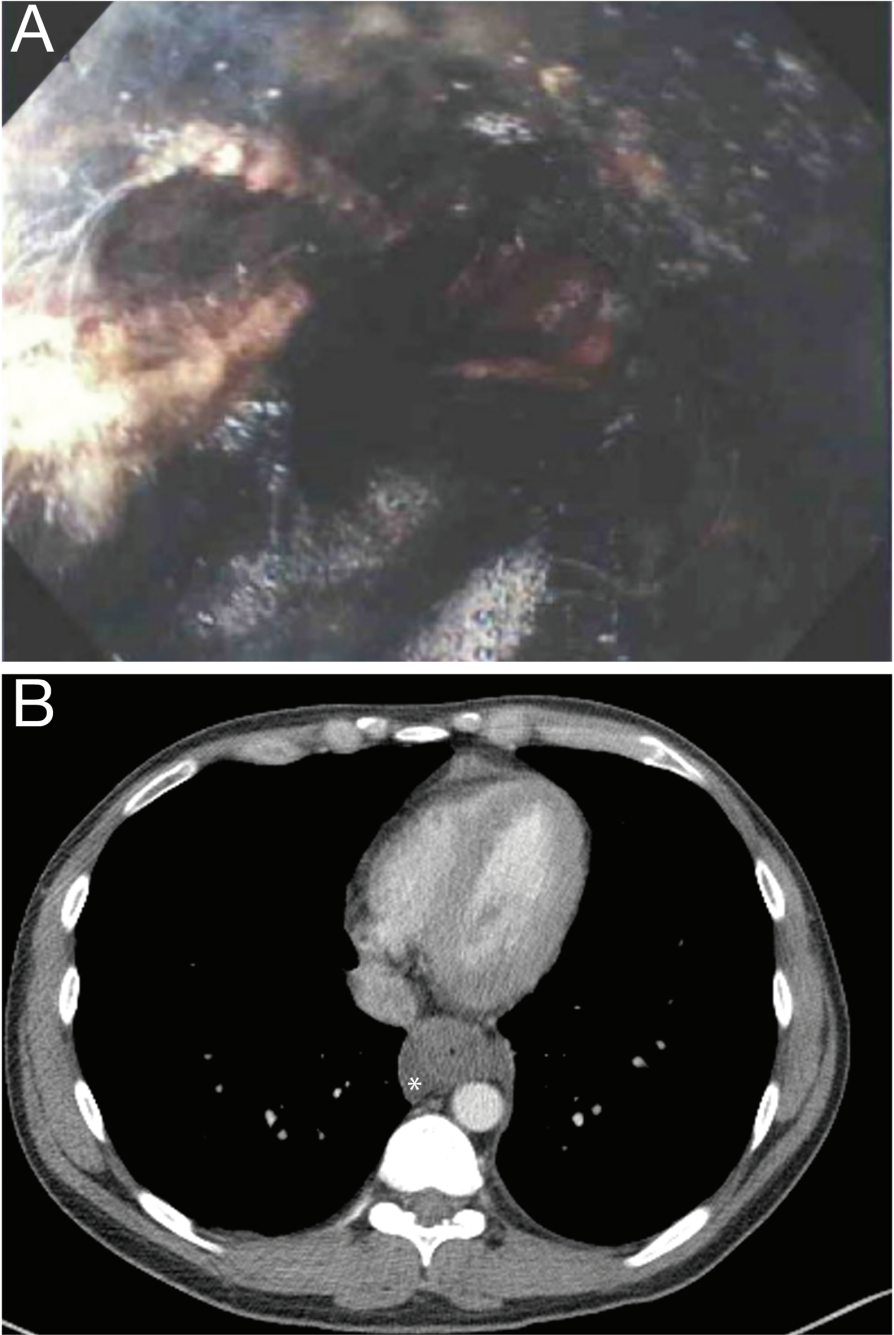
(A) Endoscopy in a patient with necrotizing esophagitis shows black mucosa. (B) CT-scan shows marked esophageal thickening and some free periesophageal fluid (asterisk).
